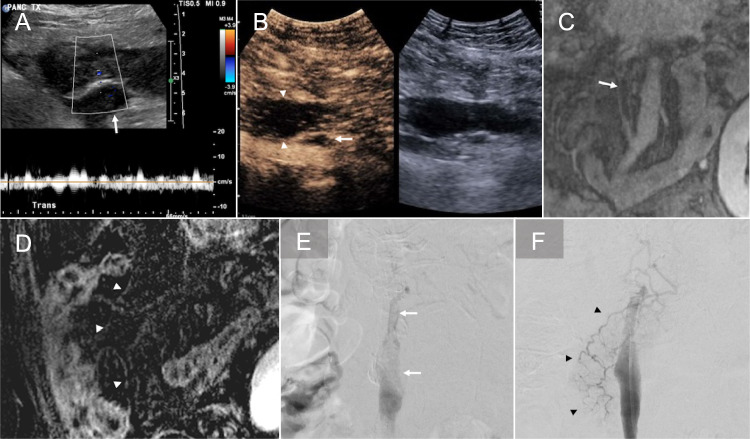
Arterial Thrombosis. A 44-year-old female with a history of type 1 diabetes mellitus status post pancreas transplant alone with elevated pancreatic enzymes. Color Doppler ultrasound (A) at the level stump of the Y graft demonstrates no arterial flow. Contrast-enhanced ultrasound (B) confirms the finding of thrombosis (arrow) as no contrast flows through the stump and no graft parenchymal enhancement (arrowhead). Magnetic resonance angiography (C) shows a filling defect in the stump of the Y arterial graft (arrow). Post-contrast magnetic resonance image (D) fat-suppressed T1-weighted imaging demonstrates loss of graft parenchymal enhancement (white arrowhead). Angiography (E) confirms an arterial thrombus as a filling defect (arrow) without parenchymal enhancement. Following percutaneous thrombectomy (F), contrast is seen in the stump of the Y graft and improved perfusion of the pancreas graft parenchyma (black arrowhead)
